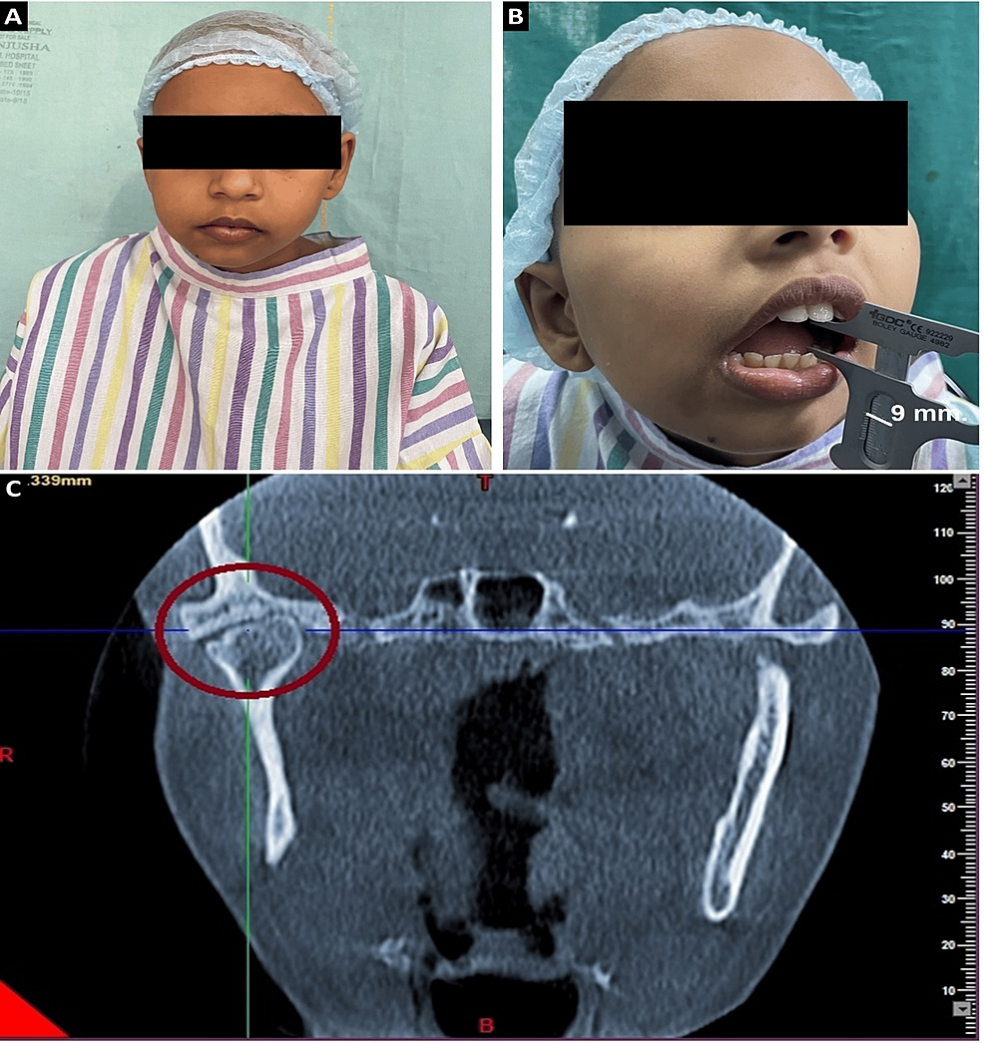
Pre-operative clinical and radiographical evaluation of the patient (A) Patient's frontal profile (B) Mouth opening limited to 9 mm with deviation of chin towards the right side (vernier caliper readings indicated by a white marker) (C) Coronal section obtained from cone beam computed tomography (CBCT), highlighting Sawhney’s Type I right temporomandibular joint ankylosis (TMJA). The ankylosed joint is delineated by a red circle for identification and reference
